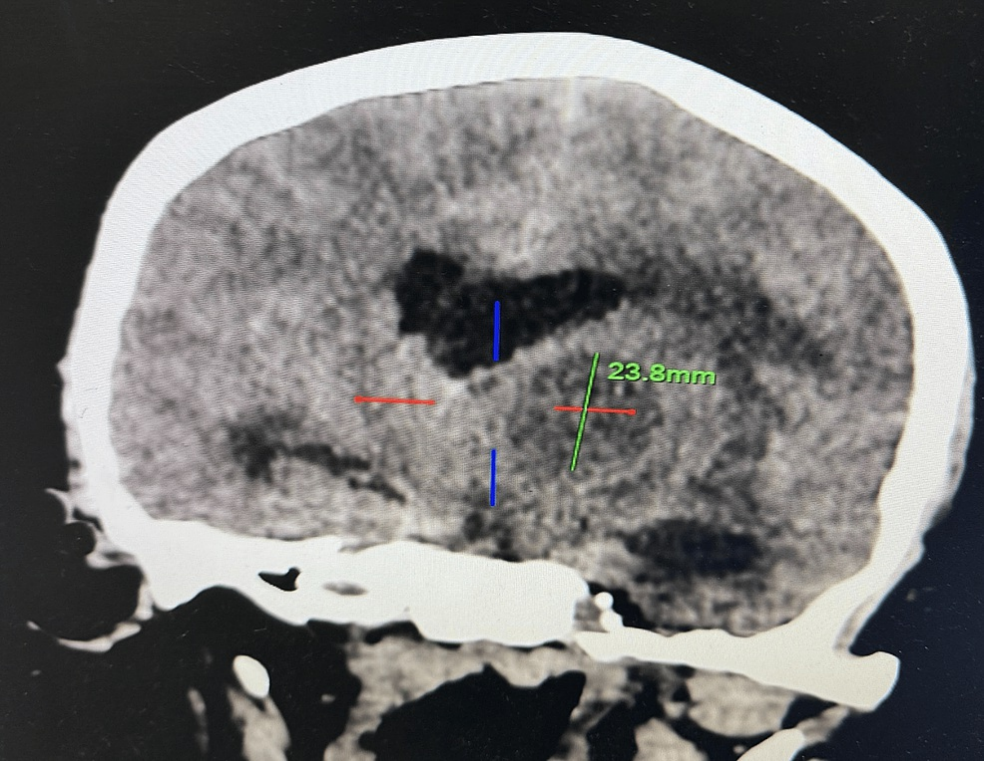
Sagittal NCCT scan showing the CC dimension of the lesion measuring 23.8 mm. The dimension of the abscess is marked with a green line: 23.8 mm in the CC direction. The red line denotes the horizontal boundaries, and the blue line represents the vertical axis through the center of the abscess. NCCT, non-contrast-enhanced computed tomography
